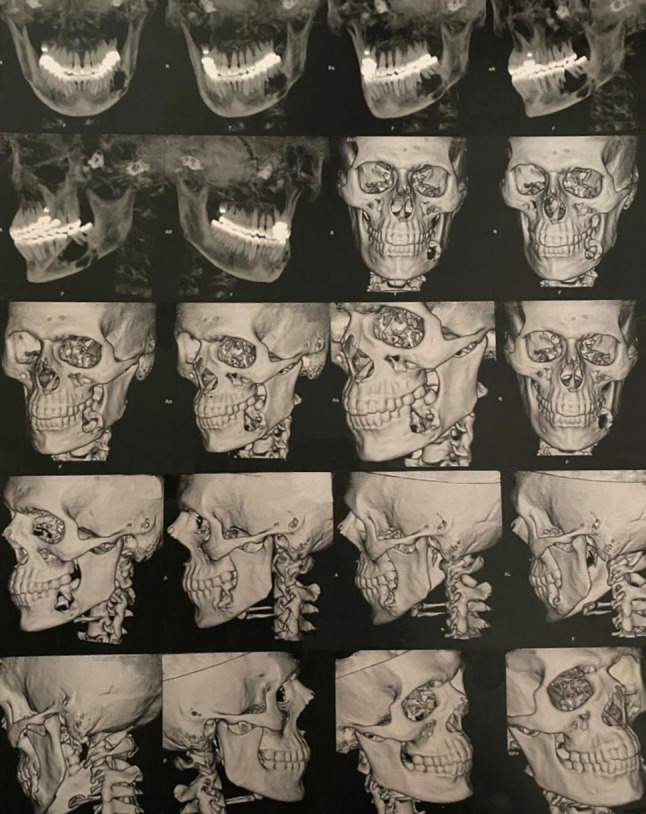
Cone beam computed tomography (CBCT) of the skull demonstrating the extent of bone destruction in the mandible
